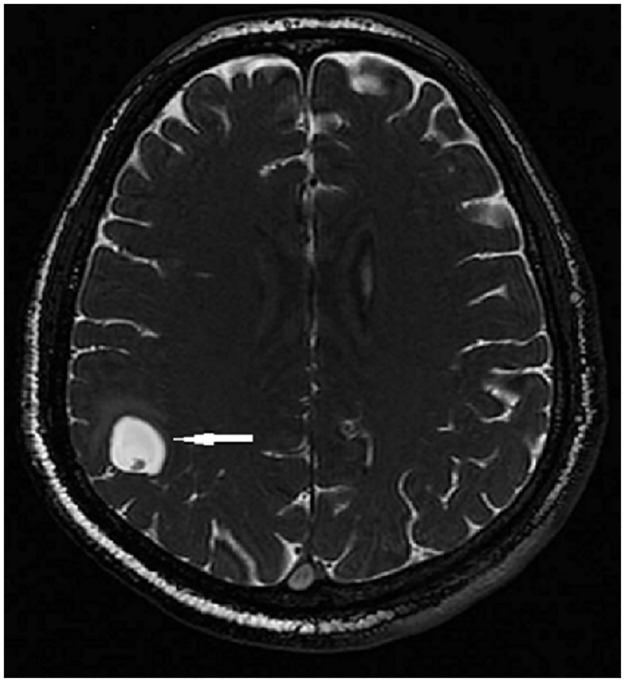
Single parenchymal cysticerci in right parietal lobe (MRI Fast Imaging Employing Steady-state Acquisition, FIESTA).
